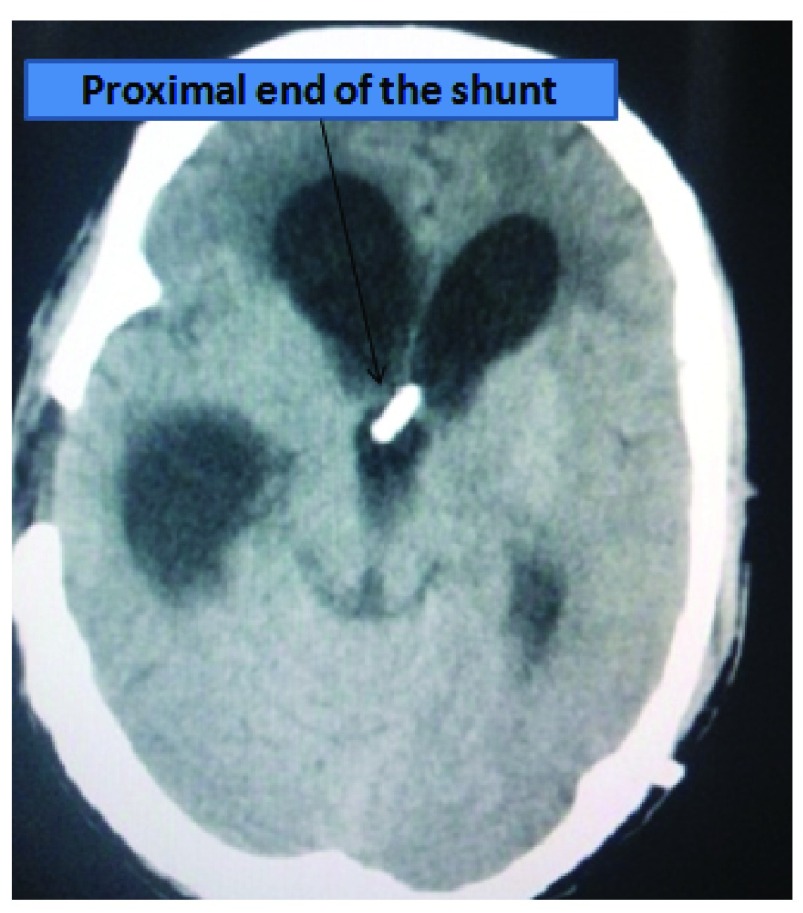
CT image confirming location of proximal end of VP shunt.
